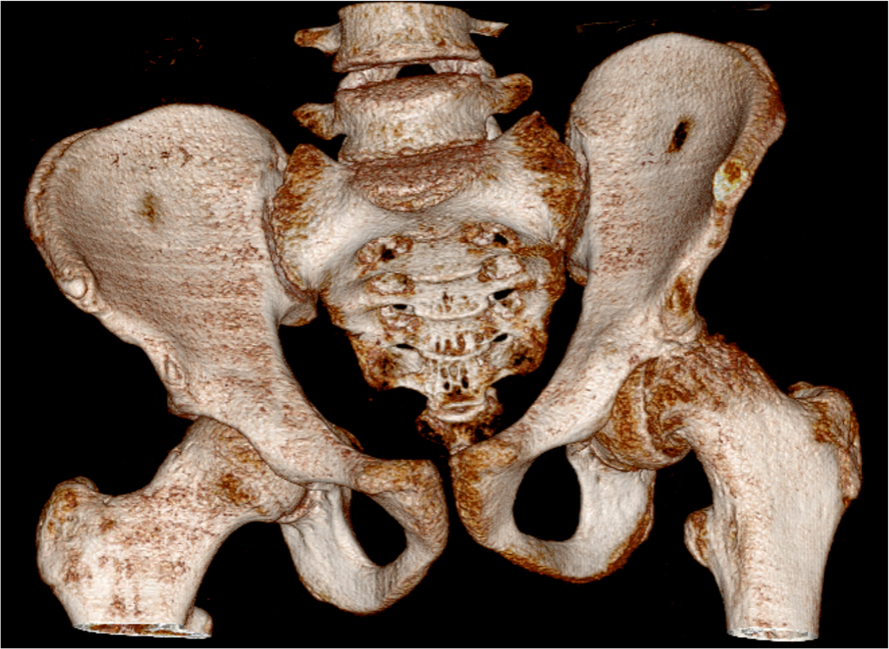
Hip CT 3D-reconstruction before surgery
